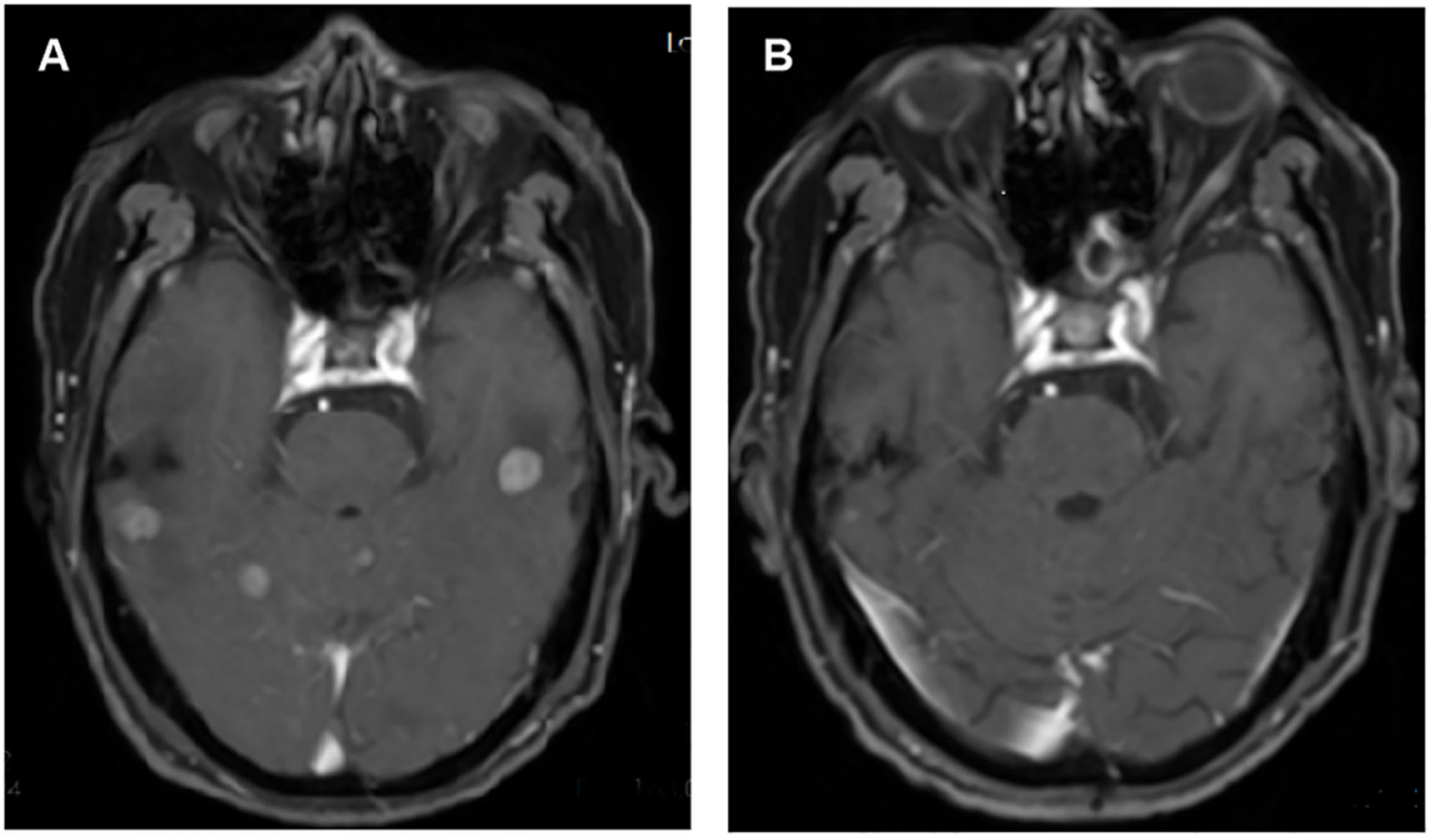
(A) Pre-treatment and (B) post-treatment of the intracranial lesion in weighted magnetic resonance images.
